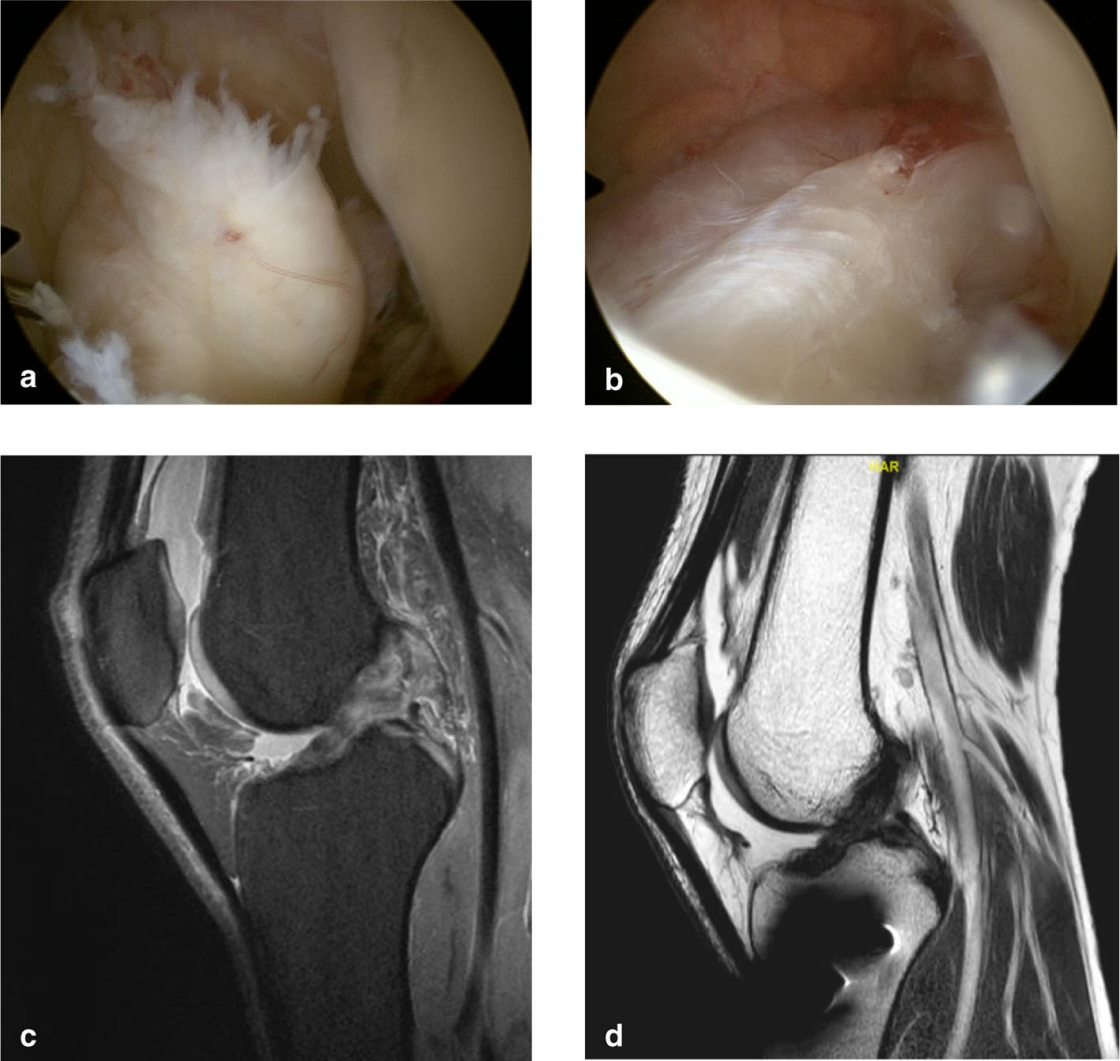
a-d, a preoperative view of the ACL tear, b preoperative view after DIS implantation, c preoperative MRI ACL tear, d postoperative MRI view with healed ACL (6 months)
